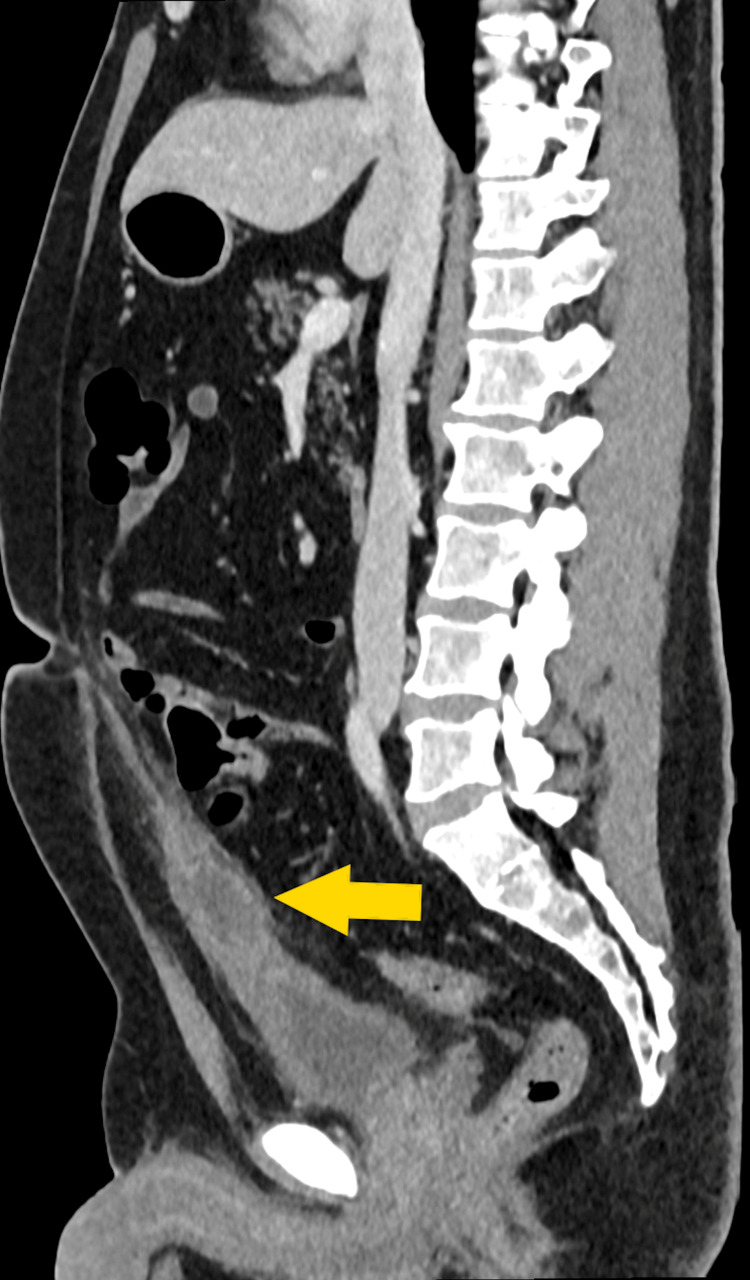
Sagittal section of the CT scan showing the urachal cyst (arrow) between the umbilicus and the bladder CT: computed tomography
